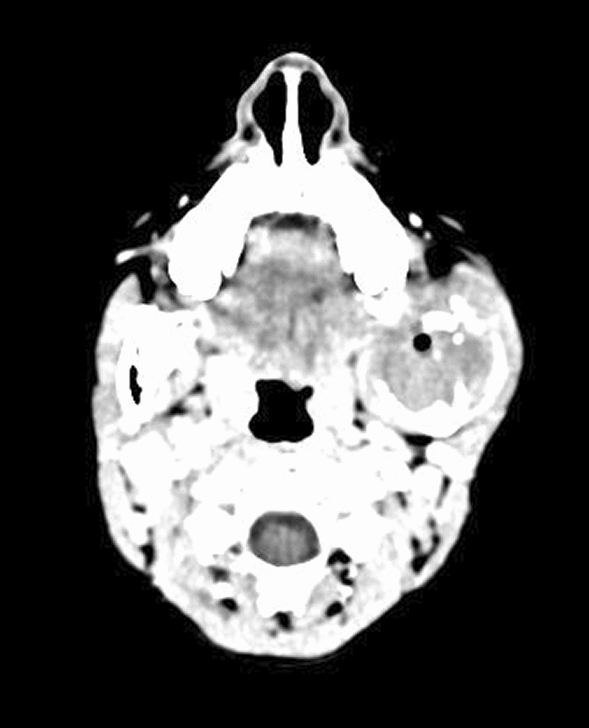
Axial section of the contrast enhanced computed tomography scan showing gross destruction of the mandible
